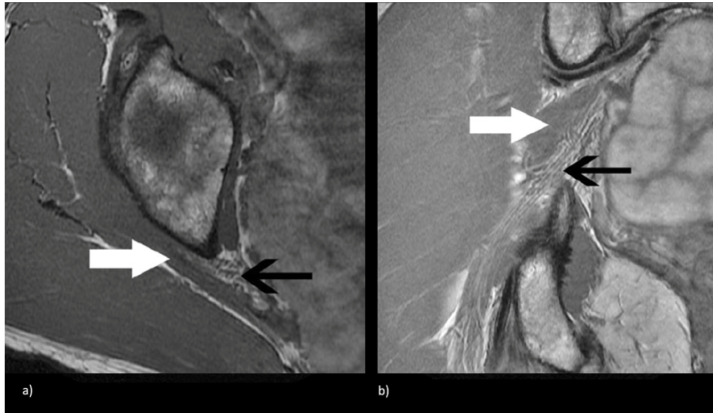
Magnetic resonance neurogram (MRN) of non-split sciatic nerve (type I). (a) Axial T1-weighted MRN of the sciatic nerve at the level of the sciatic notch demonstrates normal sciatic nerve (black arrow) anterior to the piriformis (white arrow). (b) Coronal T1-weighted sequence showing type I sciatic nerve (black arrow) inferior to the piriformis (white arrow).
